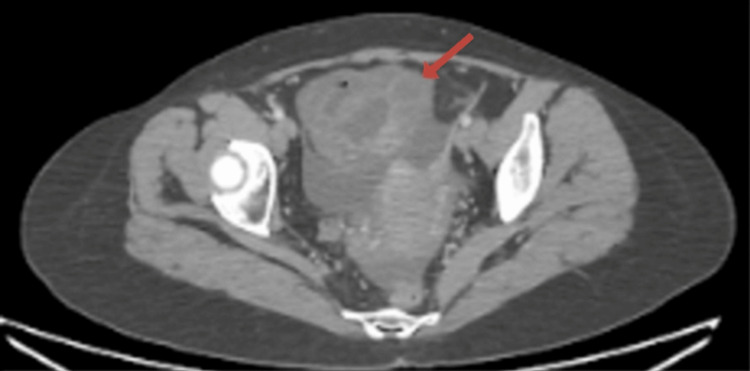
Contrast-enhanced CT of the abdomen (axial view) Circumferential thickening of the cecal wall with mucosal enhancement and submucosal edema (red arrow).
